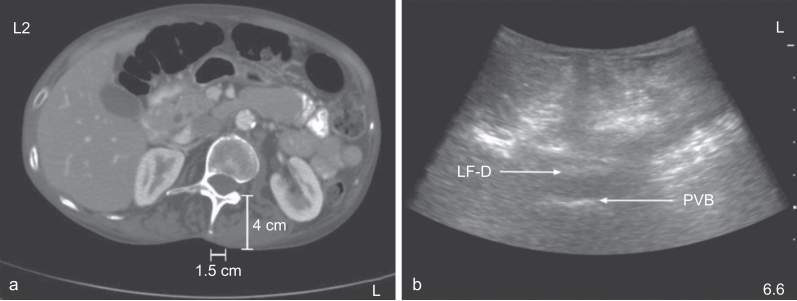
(a) Axial CT demonstrating L2 vertebral body rotation and pathway for paramedian approach to the epidural space. (b) Transverse ultrasound image depicting anatomy consistent with the CT. LF-D, ligamentum flavum–dura mater complex; PVB, posterior vertebral body.
